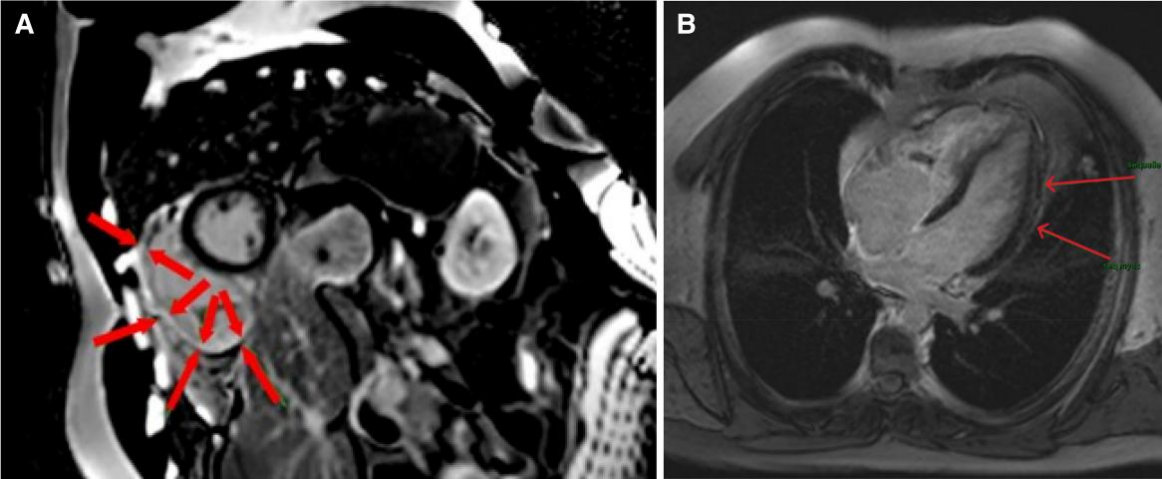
(*A*) Delayed gadolinium enhancement cardiac MRI of short axis view shows subendocardial hyper-enhancement of the right ventricle suggesting fibrosis (arrows)—(*B*) delayed gadolinium enhancement cardiac MRI shows discrete left lateral subepicardial hyper-enhancement suggesting a sequela of myocarditis.
